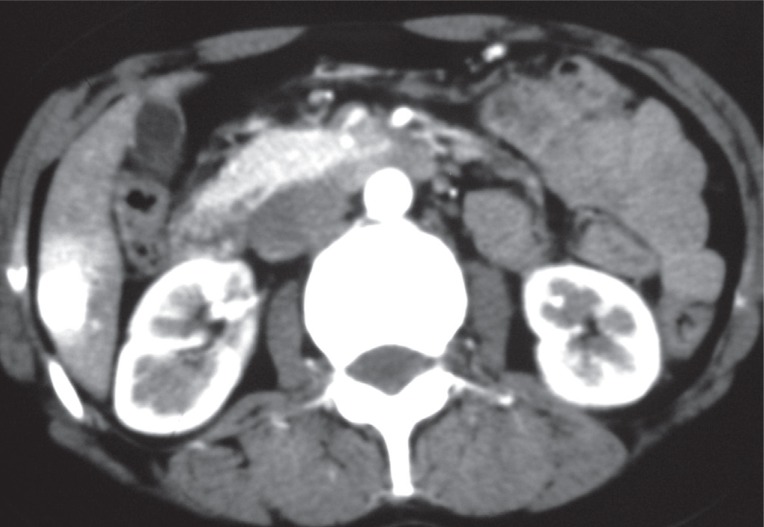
Axial CT scan after contrast injection in arterial phase. Hepatic hypervascular hemangioma with similar enhancement to the aorta and early enhancement of parenchyma adjacent to the lesion.
